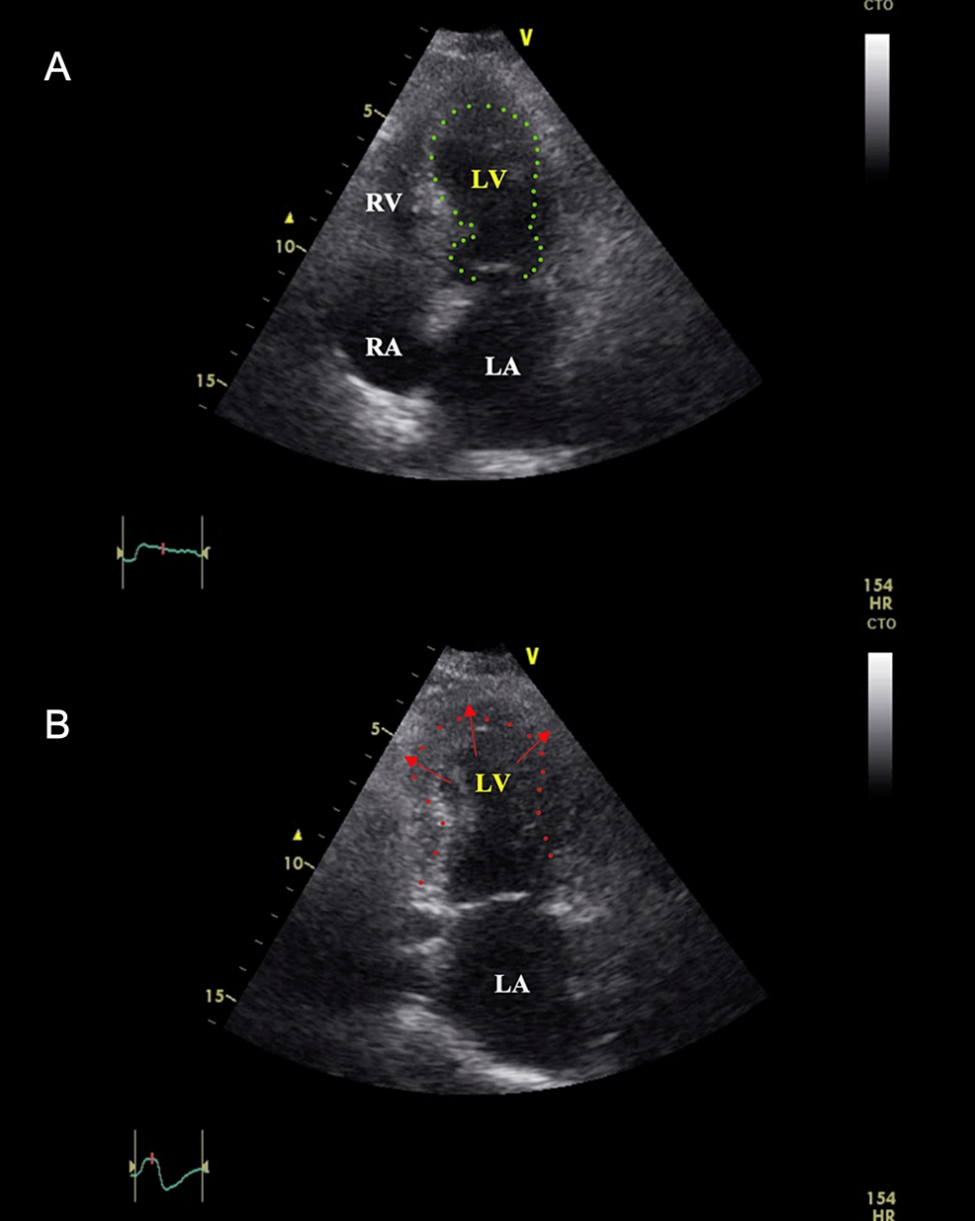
(A) Echocardiogram: apical four-chamber view showing left ventricular apical ballooning. (B) Echocardiogram: apical-two chamber view showing normal left atrium and abnormal expansion of left ventricle. Also noted, left ventricular regional wall motion abnormality-hypokinesia of mid anterior, all apical & mid segments interventricular septum.
